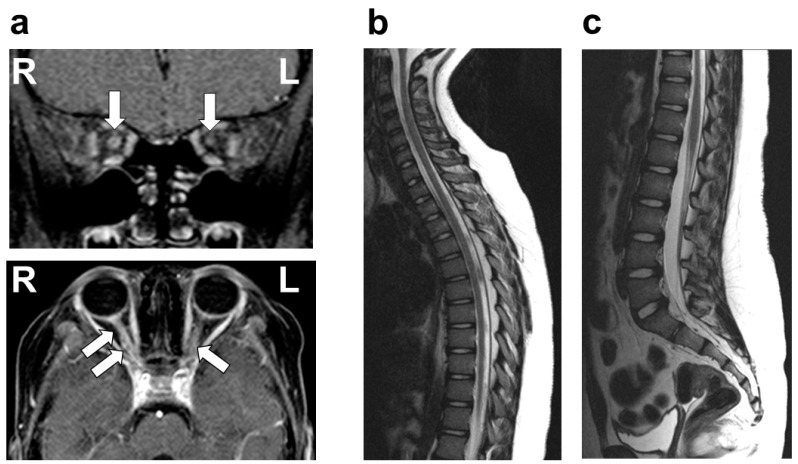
Brain and spine MRI after the first attack of optic neuritis. (a) Brain gadolinium-enhanced MRI (T1-weighted image) showing significant enhancement of the bilateral optic nerve with right-sided predominance (white arrow). The upper and lower panels represent the coronal and horizontal sections, respectively. (b,c) Thoracic spine MRI (b), and lumbar and sacral spine MRI (c), showing no abnormalities in signal intensity (sagittal T2-weighted image).
